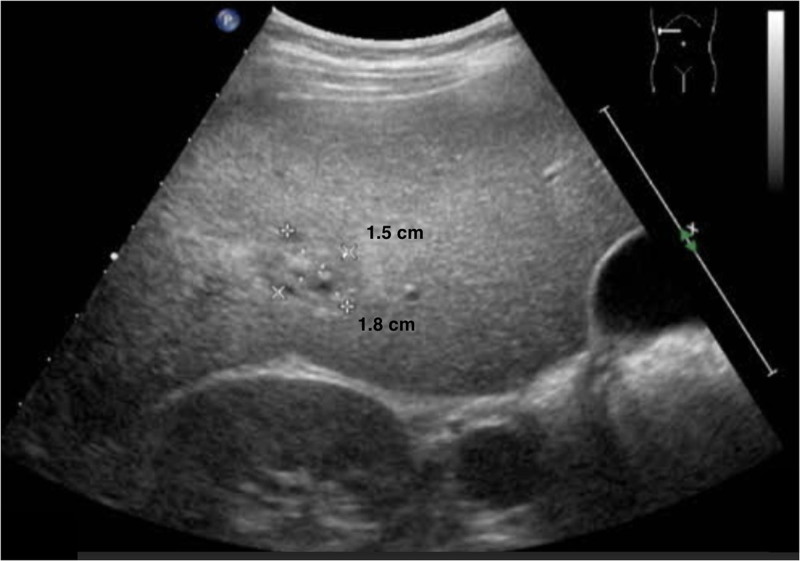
Abdominal ultrasound showing 1.8 × 1.5 cm residual lesion in liver segment 6.
